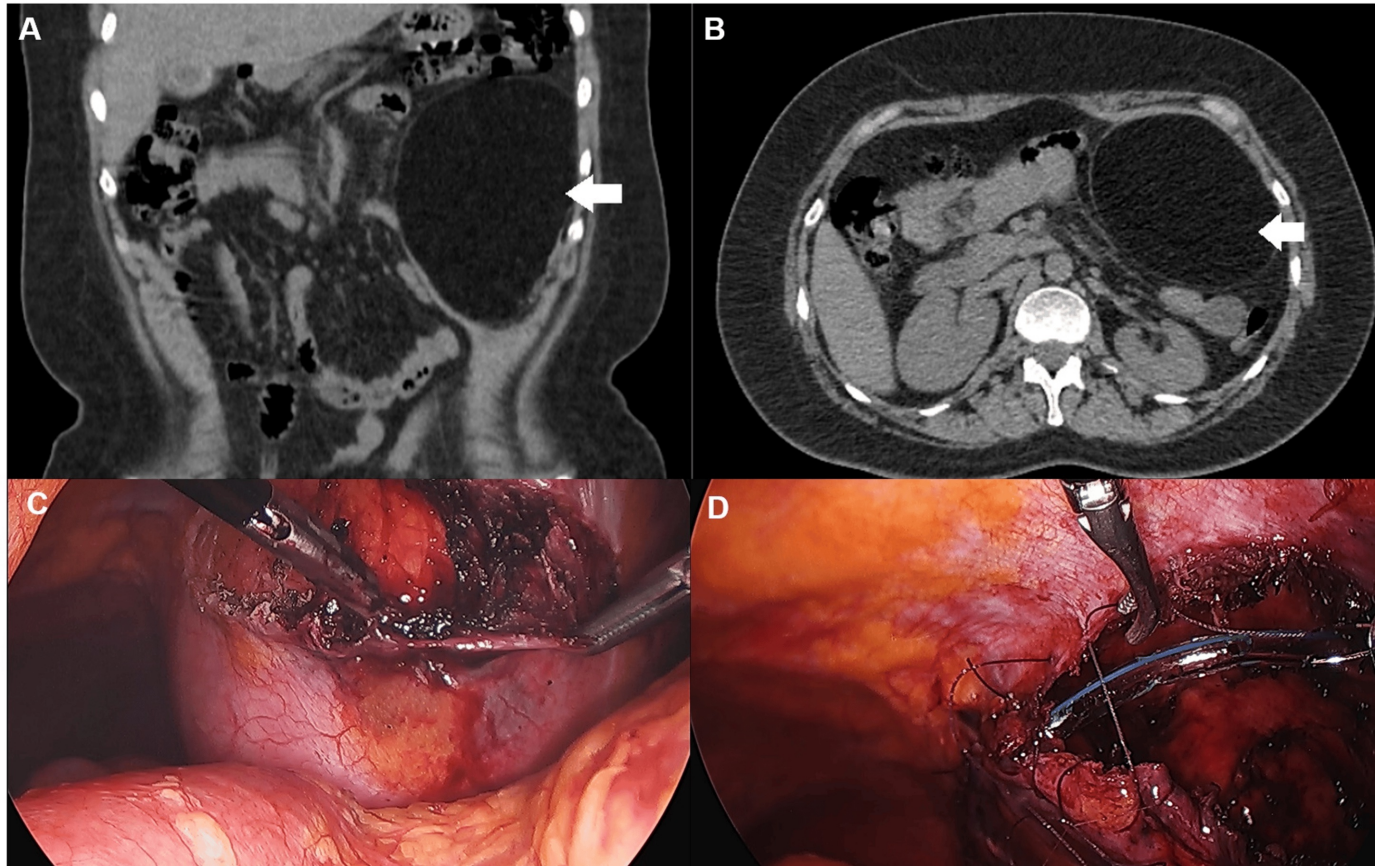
Giant parietal peritoneal lipoma (A, B) CT scan showing a large, well-defined, hypodense lesion in the parietal peritoneum (white arrows). The lesion appears homogeneous, with no significant contrast enhancement, consistent with a lipomatous tissue. (C, D) Laparoscopic images demonstrating the excision of the giant lipoma. The lesion is carefully dissected from the surrounding tissues, and the peritoneum is sutured after complete removal.
